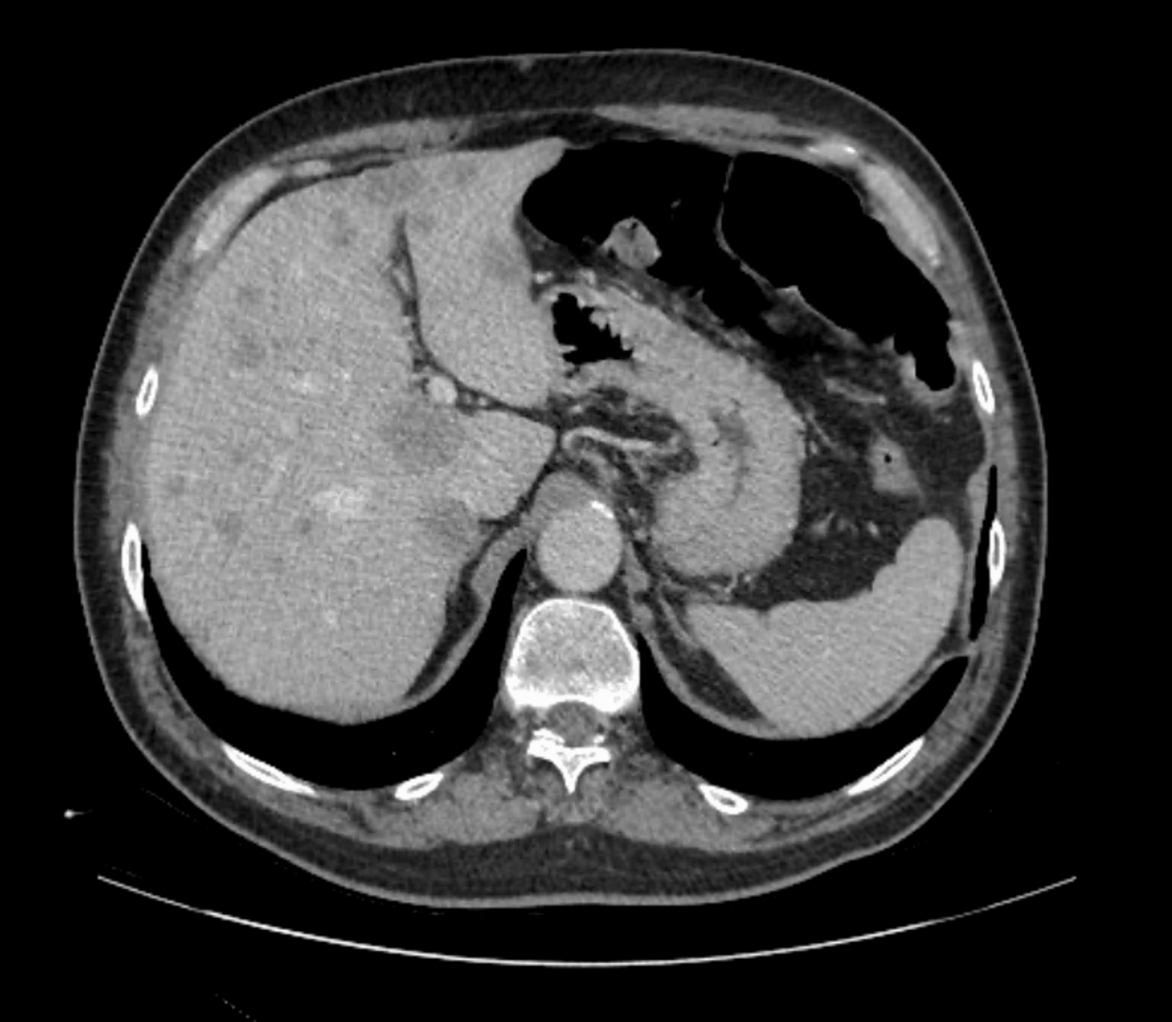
Follow-up abdominal CT after 10 months on apalutamide (Case 1) New metastatic hepatic lesions.
